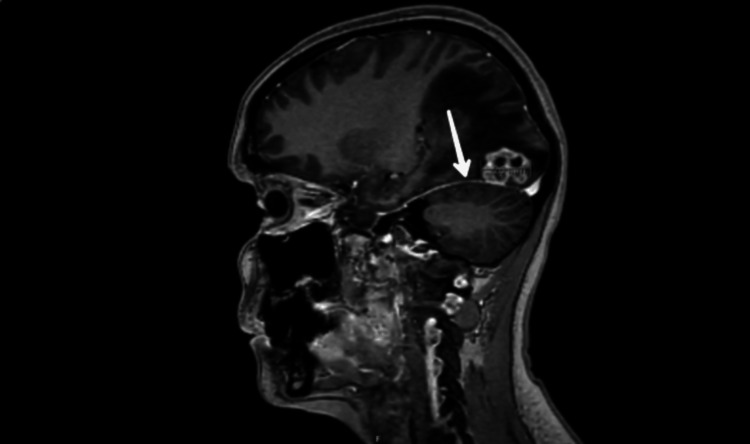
MRI of the head with contrast sagittal section demonstrating evidence of cerebellar surface deposits in keeping with leptomeningeal disease. MRI: magnetic resonance imaging
